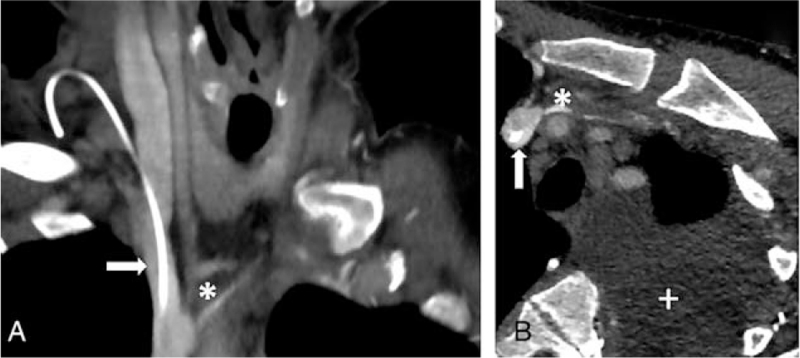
Injected CT scan performed at admission (A: coronal reconstruction, B: corrected axial reconstruction). The thrombus within the left brachiocephalic vein is well individualized (∗), ending at the junction between this vein and the superior vena cava (arrow). Presence of a large pleural effusion, a chylothorax in our case (+).
